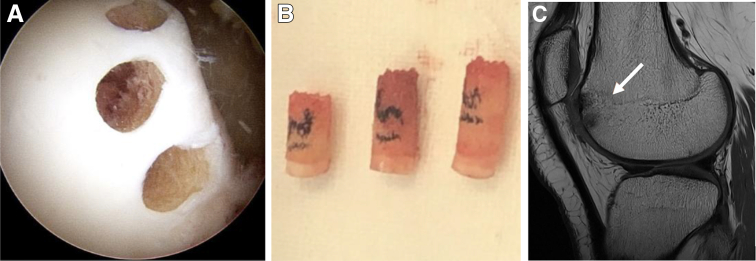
(A) Patient in the supine position. Arthroscopy of the left knee through the anterolateral portal, with view of the harvest site of 3 osteochondral autograft plugs from the lateral trochlea. (B) Intraoperative image of 3 osteochondral autograft plugs of various lengths visualized on the prep table following harvest, ready for reimplantation to the site of the osteochondritis dissecans lesion. (C) Sagittal T1-weighted magnetic resonance imaging of the mosaicplasty donor site showing restoration of bone and cartilage postoperatively.
